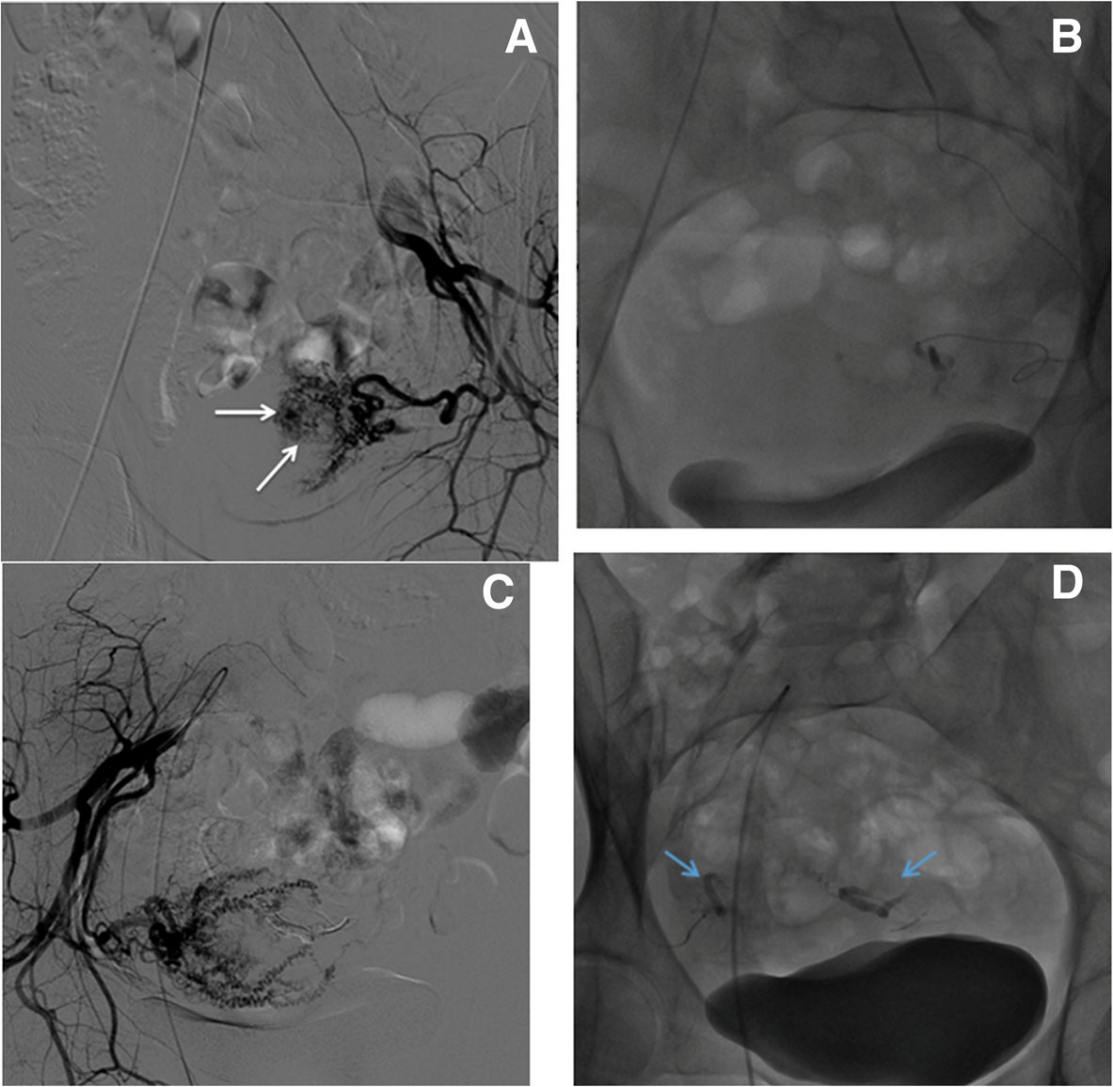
Arteriovenous Malformation (AVM) embolization. a Right transfemoral DSA of the left hypogastric artery shows an uterine AVM (white arrows). b A superselective embolization with microcatheter of the left uterine artery is performed using PVA particles and Squid-18. c Diagnostic DSA of the right hypogastric artery. d After bilateral uterine AVM embolization, X-rays show both uterine arteries opacified with Squid (light blue arrows)
